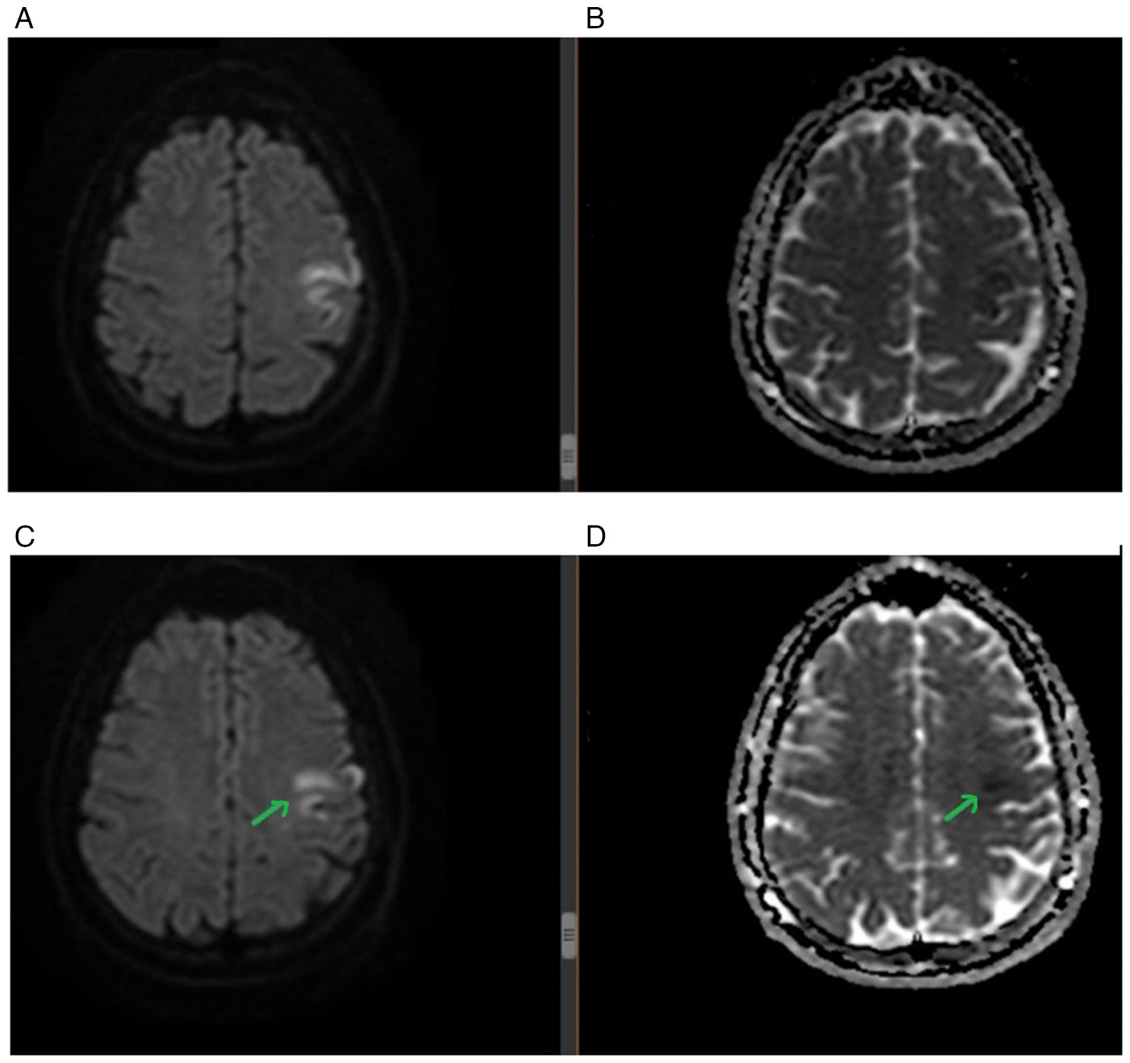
MRI findings. (A) Hyperintense lesion in diffusion sequence at the left frontal level. (B) Low apparent diffusion coefficient correspondence at the left frontal level. (C) Hyperintense lesion in diffusion sequence affecting the middle gyrus and precentral gyrus on the left side (green arrow). (D) Low apparent diffusion coefficient correspondence at the middle gyrus and the precentral gyrus on the left side (green arrow).
